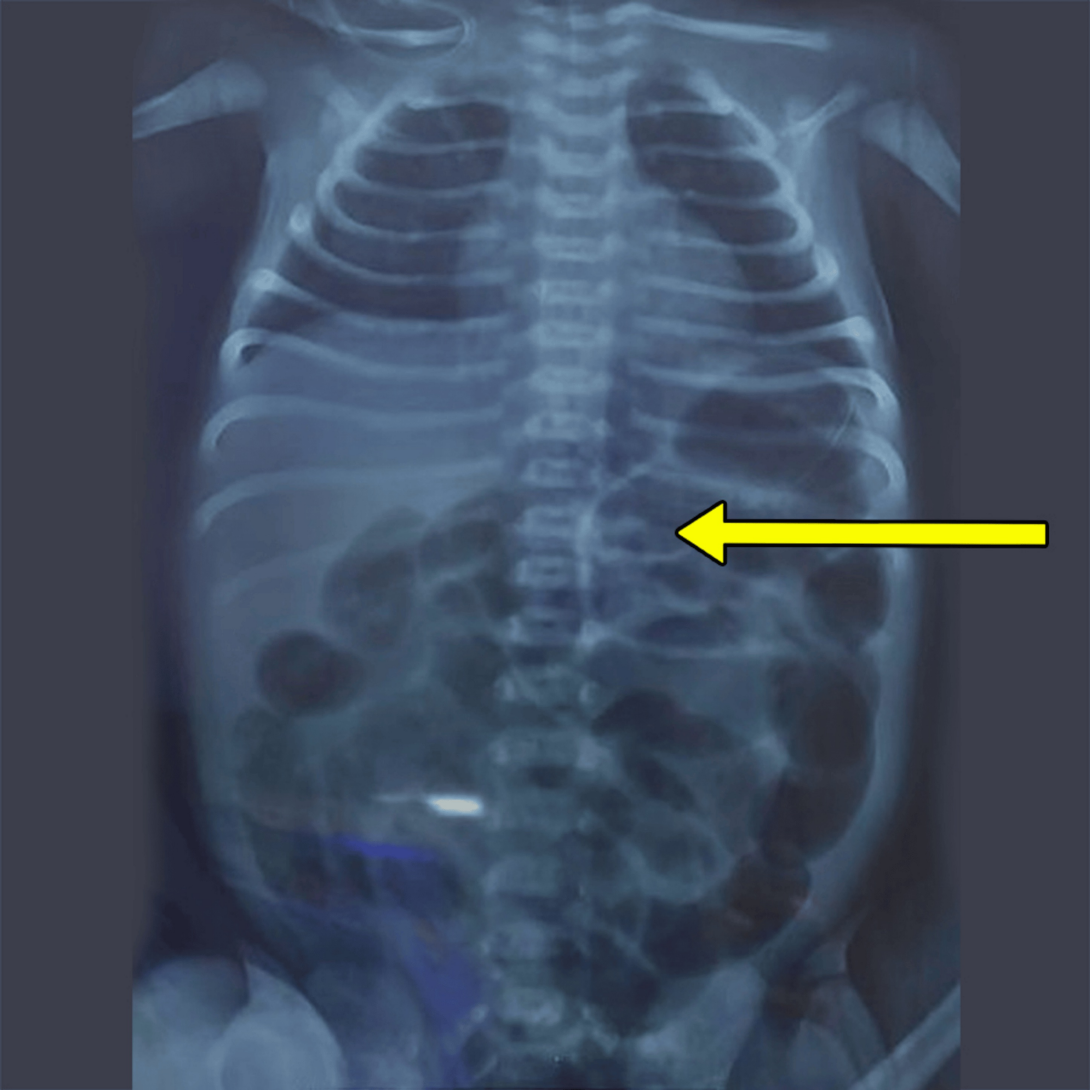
X-ray of the abdomen and pelvis showing a normal bowel gas pattern (yellow arrow)
